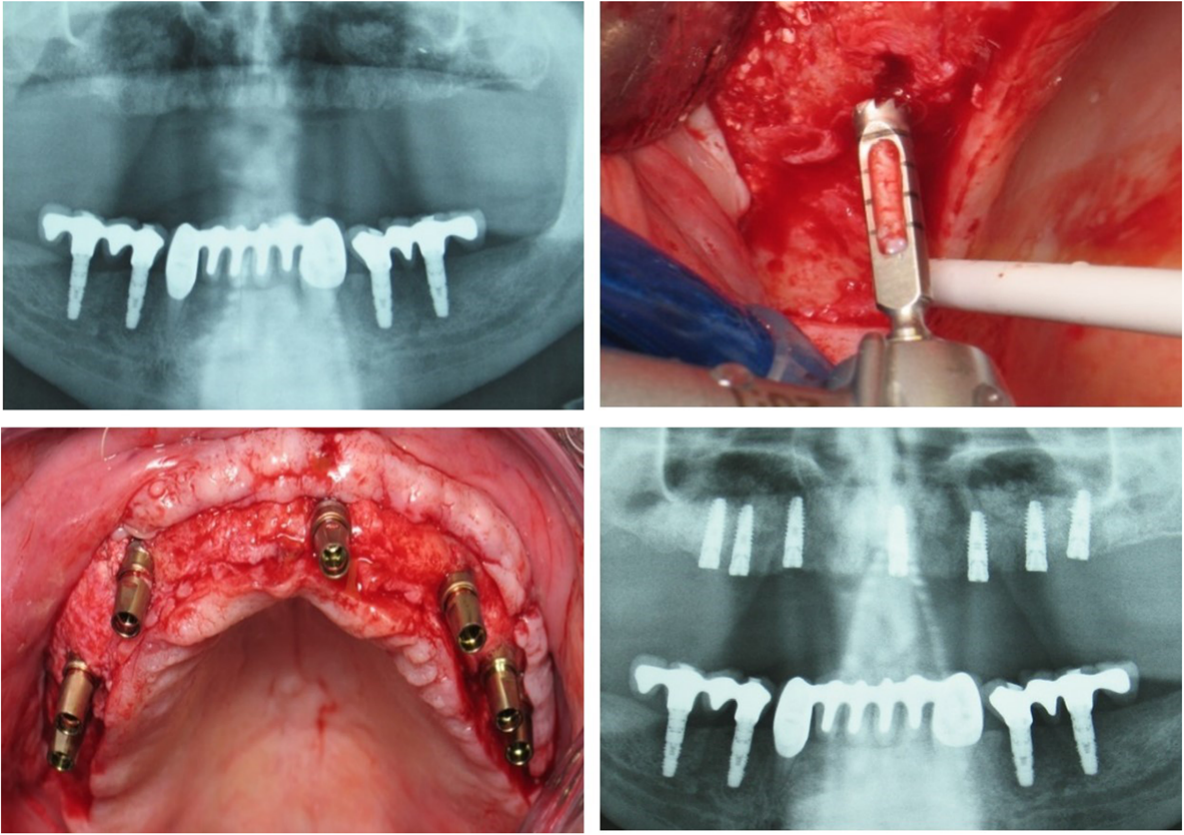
Panoramic X-ray of the case presented in Fig. 2, 6 months post-operatively (a), showing increased radio-opacity in both grafted sinuses. Biopsies were obtained with trephine burs during oral implant site preparation (b)
